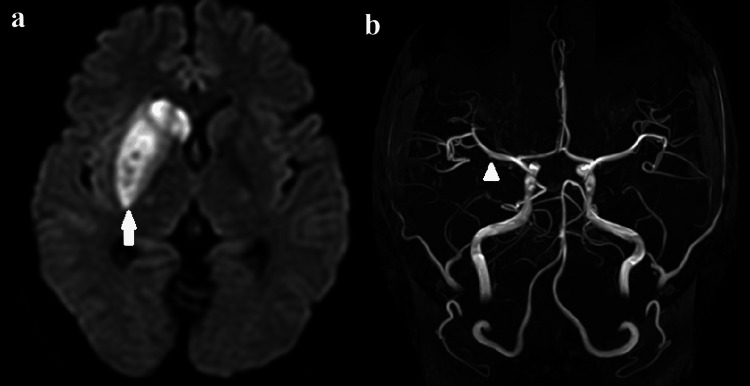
Postoperative MRI shows (a) intensified high-intensity area without any high area on the cortex after the endovascular sugery and (b) clear delineation of right middle cerebral artery
